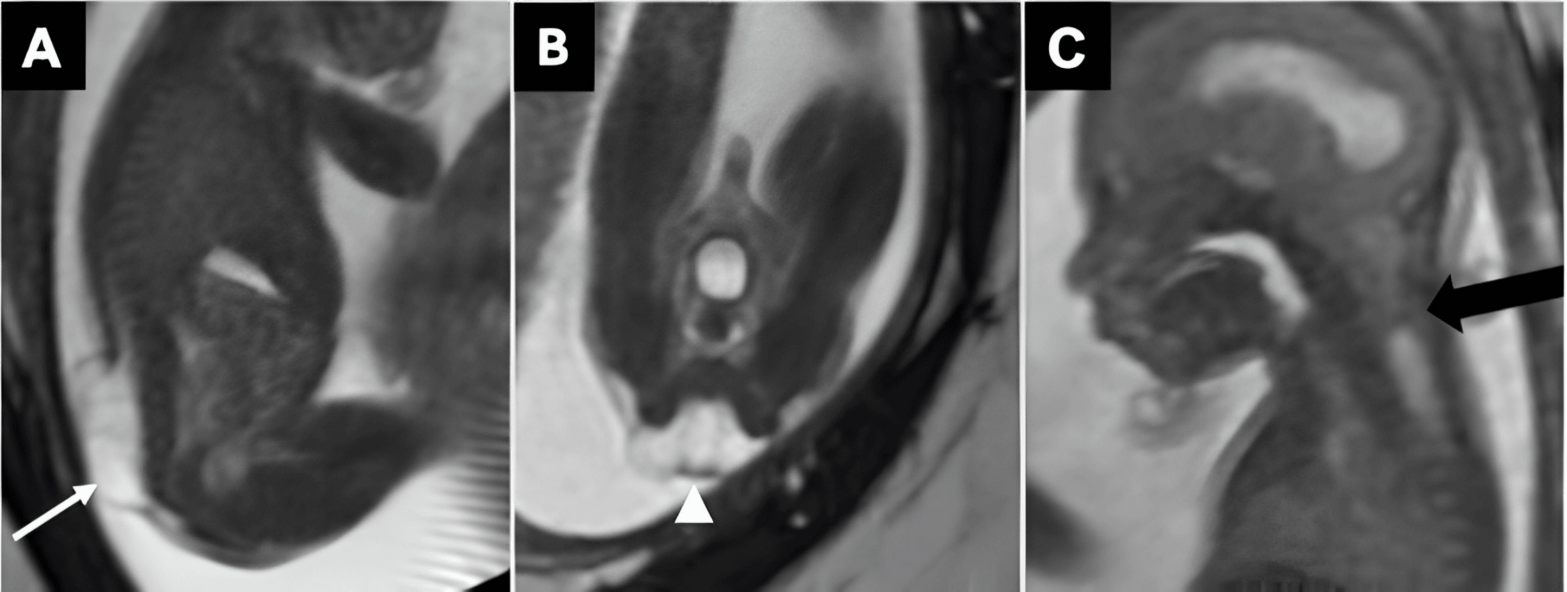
Sagittal (A) and coronal (B) T2-weighted fetal MR images of a myelomeningocele demonstrate a dorsal spinal defect not covered by skin (white arrow) and extension of the neural placode (white arrowhead) beyond the skin surface. Sagittal T2-weighted fetal MR image of the brain and upper cervical spine (C) reveals a small posterior fossa with cerebellar tonsillar herniation (black arrow), one of the features of a concomitant Chiari II malformation. All images were obtained by the authors from the institutional picture archiving and communication system (PACS). They have been de-identified and used in compliance with institutional policies.
